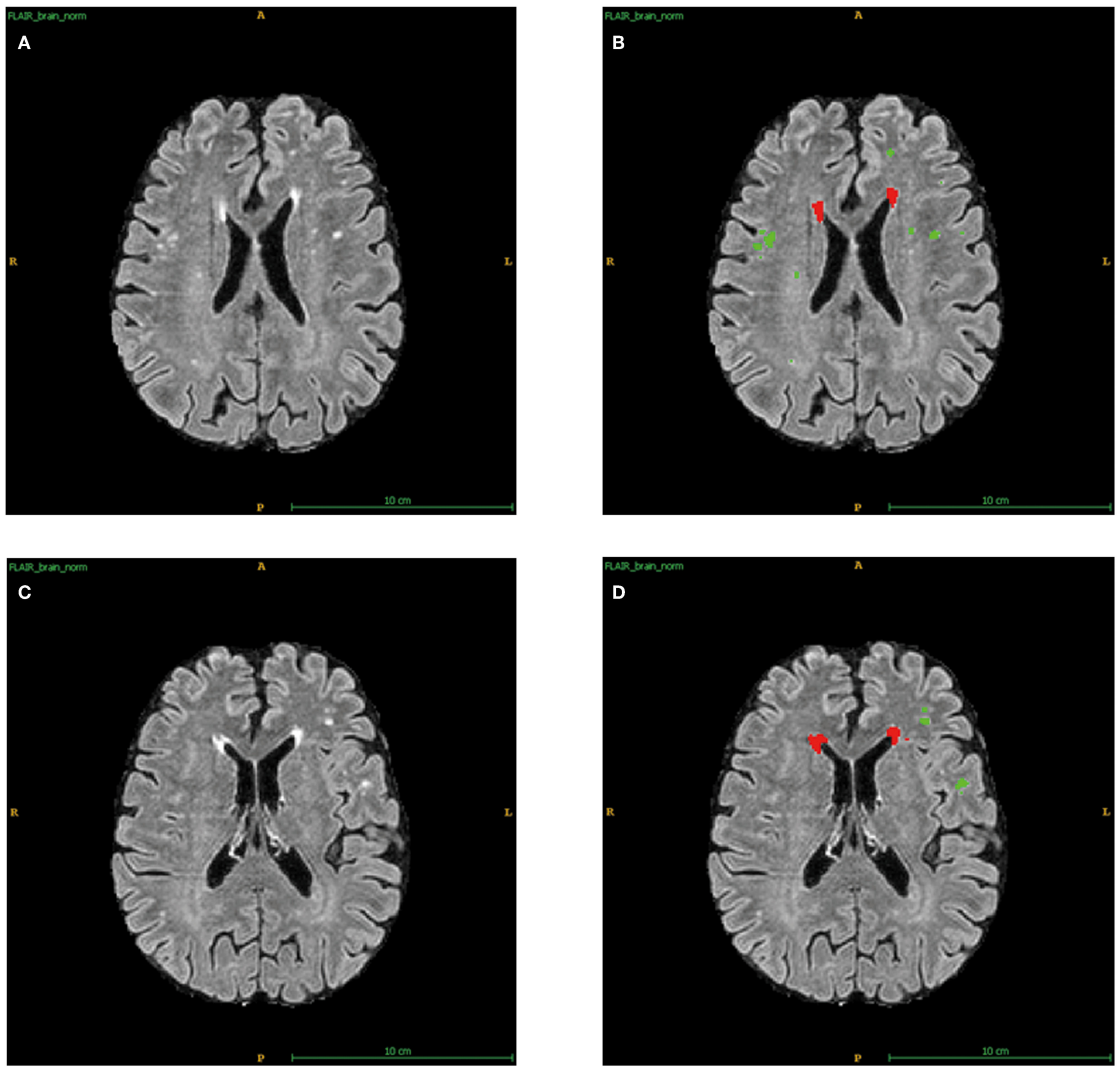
Automatic segments of PVWMHs and DWMHs applied by AccuBrain^®^. (A) The original T2-FLAIR images of sample 1. (B) Automatic division of PVWMHs (Red) and DWMHs (Green) for (A). (C) The original T2-FLAIR images of sample 2. (D) Automatic division of PVWMHs (Red) and DWMHs (Green) for (C).
